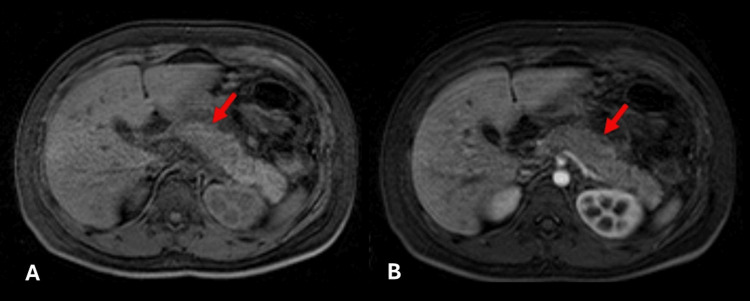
Contrast-enhanced MRI (abdomen): Axial T1-weighted sequence (A) shows increased pancreatic thickness and heterogeneous hypointense signal (red arrow). Arterial phase contrast-enhanced axial T1-weighted sequence (B) shows hypointense pancreatic signal (red arrow).
